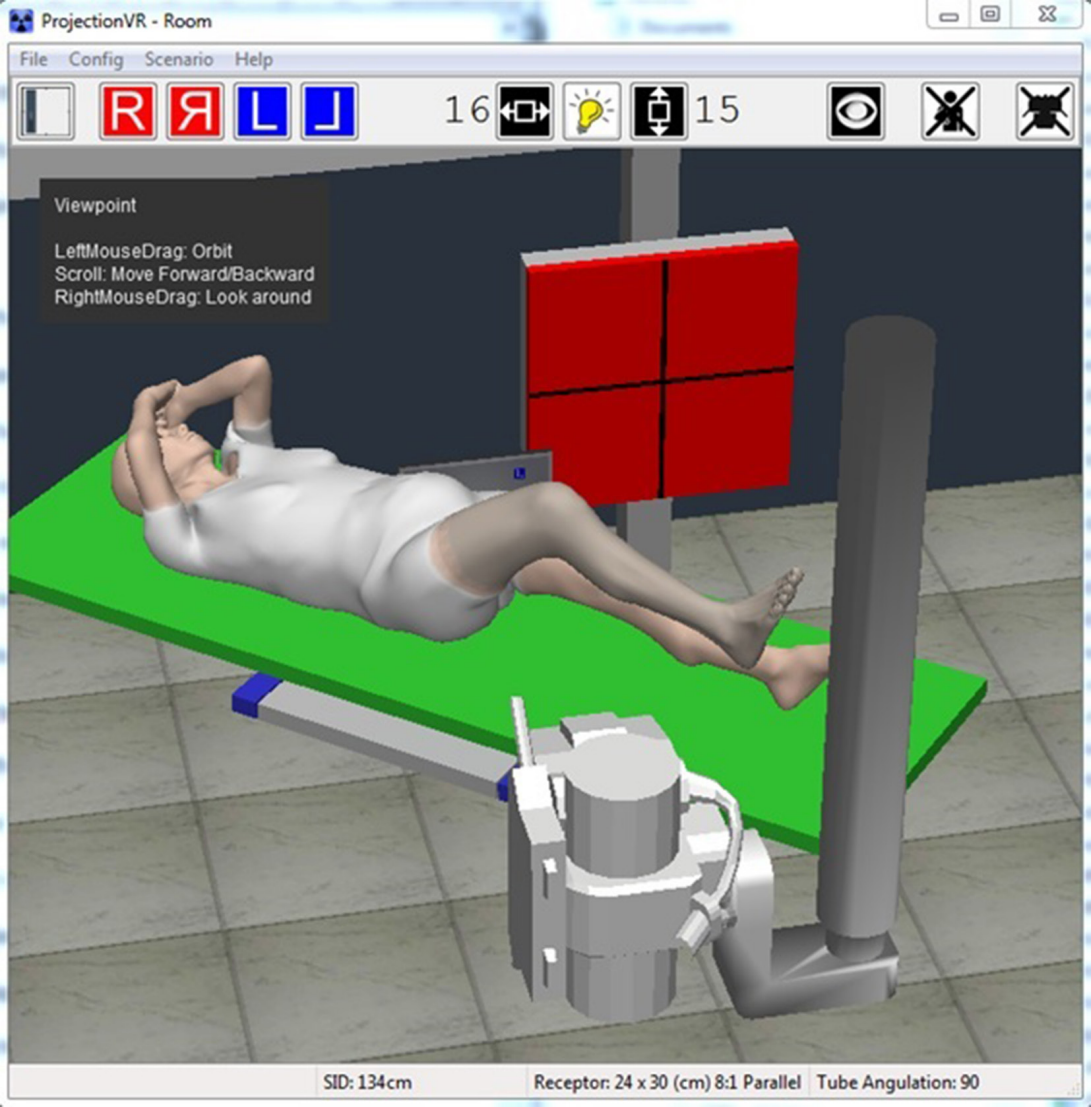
ProjectionVR^TM^ (v. 5.0) X-ray room interface.
